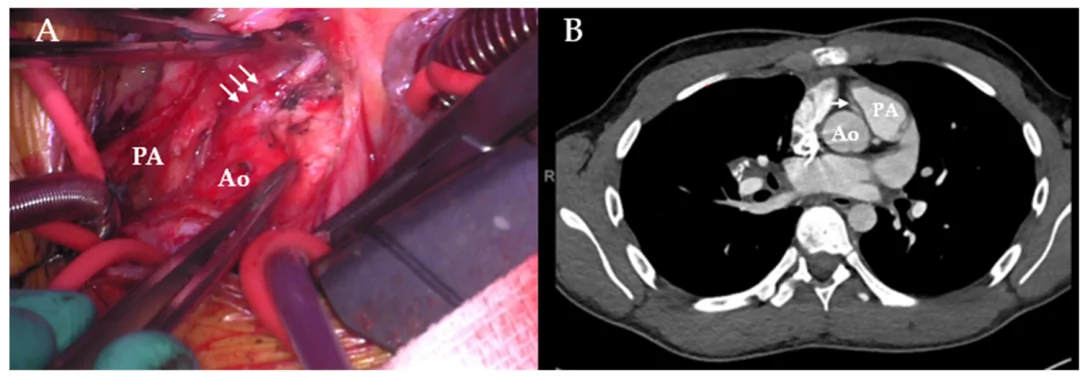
(A) intraoperative photo showing the right coronary artery (multiple white arrows) running an inter-arterial course between the aorta and the main pulmonary artery, (B) preoperative computed tomography scan demonstrating the same course. In this case, there is no intramural segment; (Ao: ascending aorta; PA: pulmonary artery).
